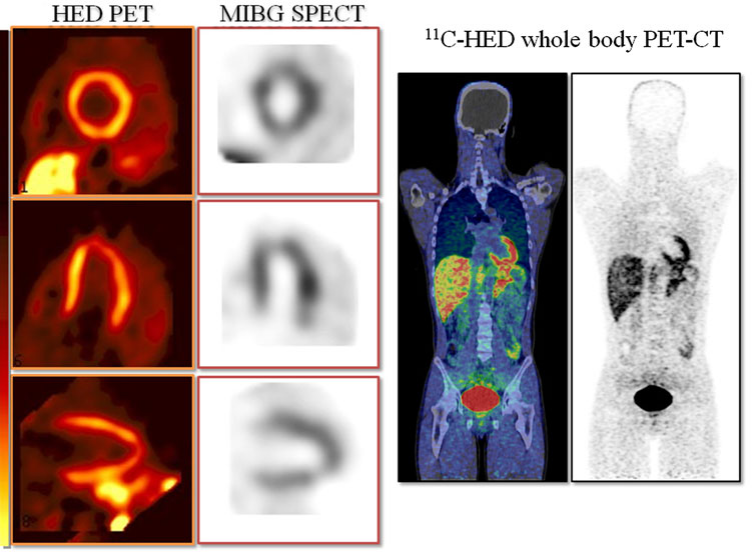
^11^C-hydroxyephedrine (HED) PET and MIBG SPECT of a normal subject. The HED PET obtained better-quality images in the myocardium as compared to the MIBG SPECT (Color figure online)
